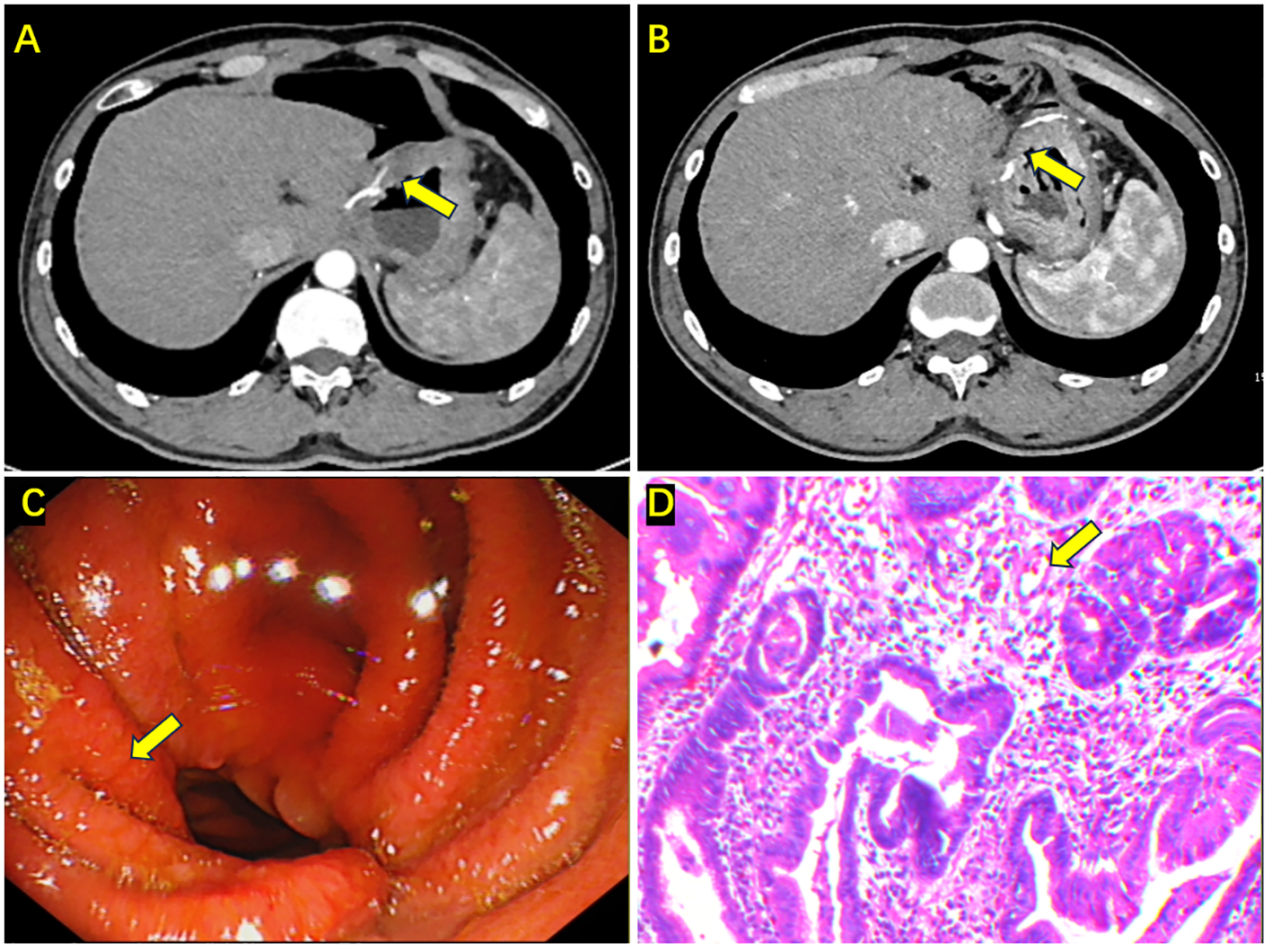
Enhanced abdominal CT, gastroscopy, and biopsy results after completing all treatments. (A, B) Enhanced CT shows edema and thickening at the anastomosis, with no obvious enhancement (the yellow arrow); (C) Gastroscopy showing congestion and edema at the anastomosis, with a rough mucosa (the yellow arrow); (D) Biopsy indicating chronic mucosal inflammation, epithelial hyperplasia, interstitial edema, and no apparent tumor cells in the tissue (the yellow arrow).
